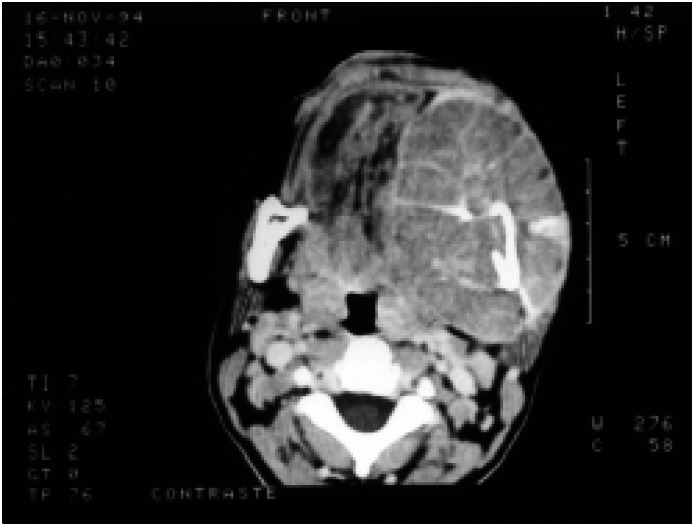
CT scan of a capsulated mass involving the mandible with septa and deviation of the surrounding tissues.
